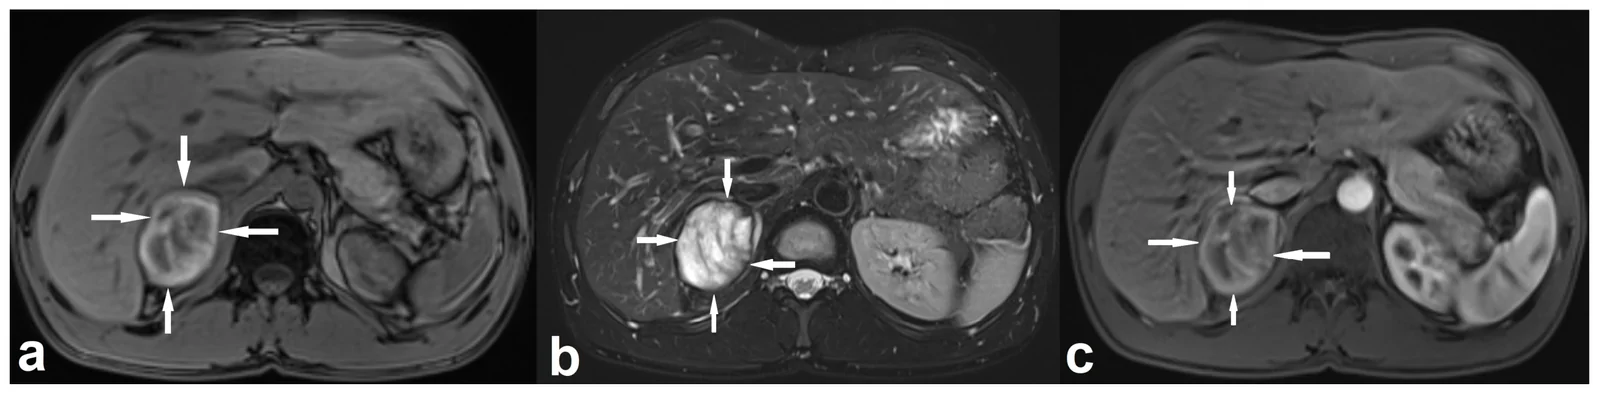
Axial MRI images: (a) T1-weighted pre-contrast image, (b) fat-suppressed T2-weighted image, and (c) T1-weighted post-contrast arterial-phase image demonstrating the heterogeneous right adrenal hemorrhagic lesion (arrows).
